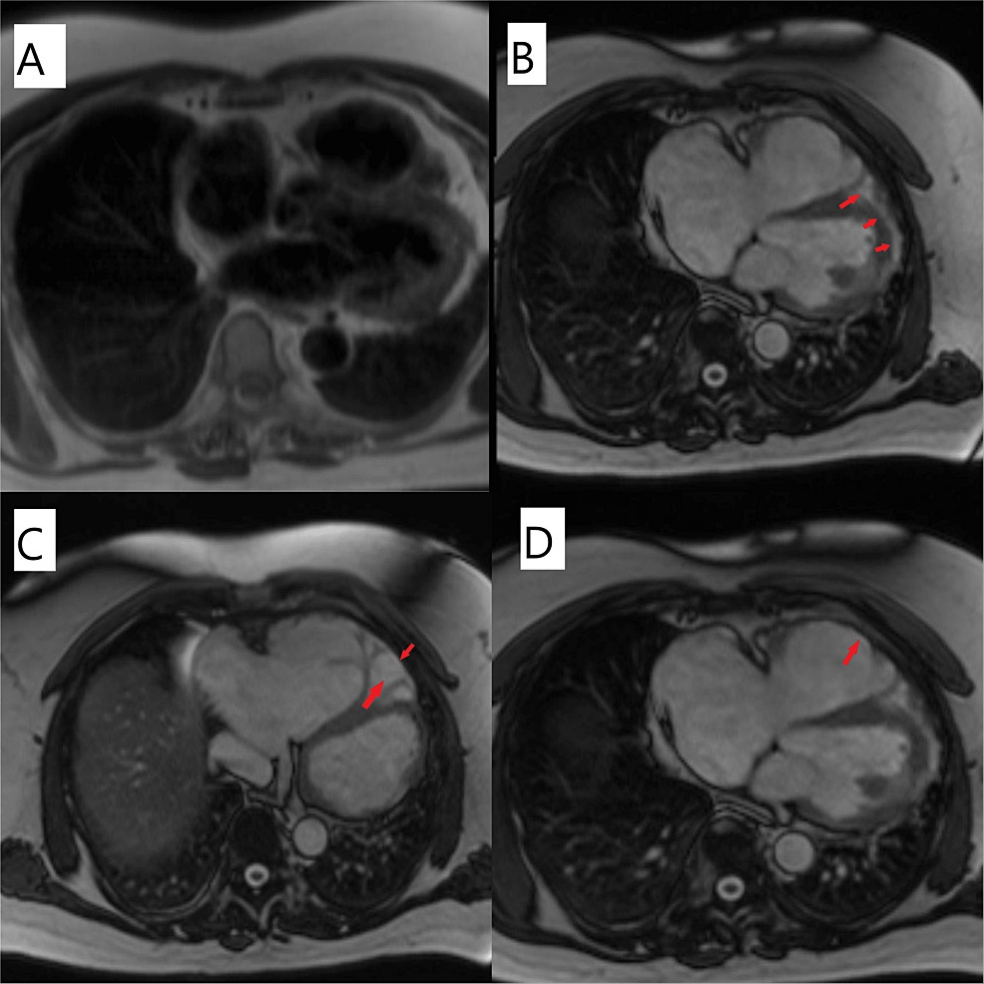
Cardiac magnetic resonance imaging (MRI) showing ARVC A - Cardiac MRI picture in diastole; B - Cardiac MRI showing the parasternal long-axis view. Red arrows show areas of inferolateral sub-epicardial left ventricular enhancement in the late Gadolinium series; C and D - Cardiac MRI showing the parasternal long-axis view. Red arrows show right ventricular global transmural fibrofatty infiltration and extensive scarring, in the late Gadolinium series. ARVC: arrhythmogenic right ventricular cardiomyopathy
